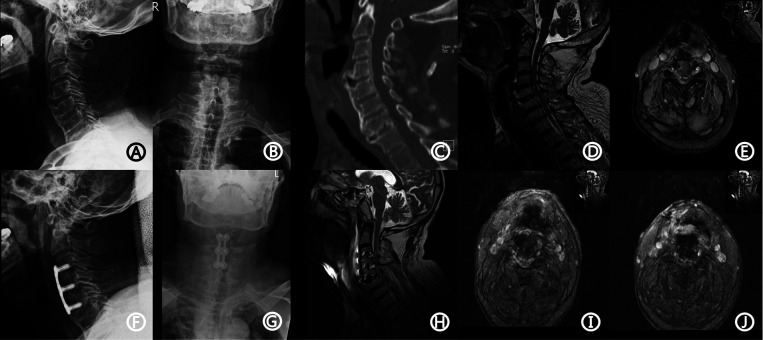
The patient's imaging data. Preoperative x-ray showed degenerative changes (A,B). CT showed a bone bridge in the anterior part of the vertebral body (C). MRI showed disc herniation at the level of C3/4 and C4/5 vertebrae, causing compression of the spinal cord and corresponding spinal canal stenosis (D,E). Postoperative x-ray showed the good position of the plate and interbody (F,G). Emergency MRI showed low T1 and strip long T2 signal located behind C3/4 and C4/5 discs, and DPOSEH was suggested (H–J). CT, computed tomography; MRI, magnetic resonance imaging; DPOSEH, delayed postoperative spinal epidural hematoma.
